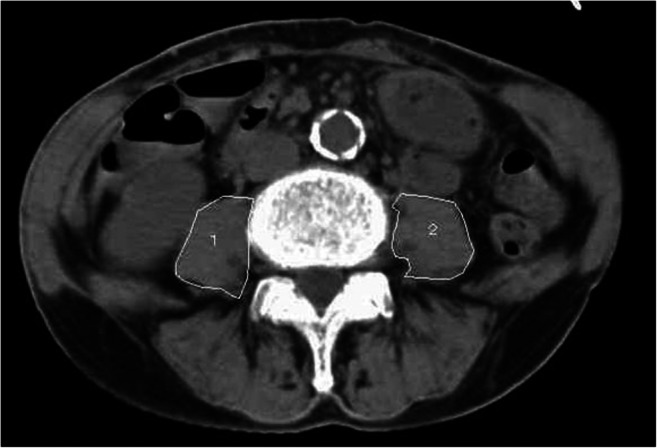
Measurement of the psoas muscle area on preoperative CT. Cross-sectional areas (mm^2^) of the psoas muscle at the level of the inferior border of the fourth lumbar vertebra (L4) measured by manual tracing on CT scans
